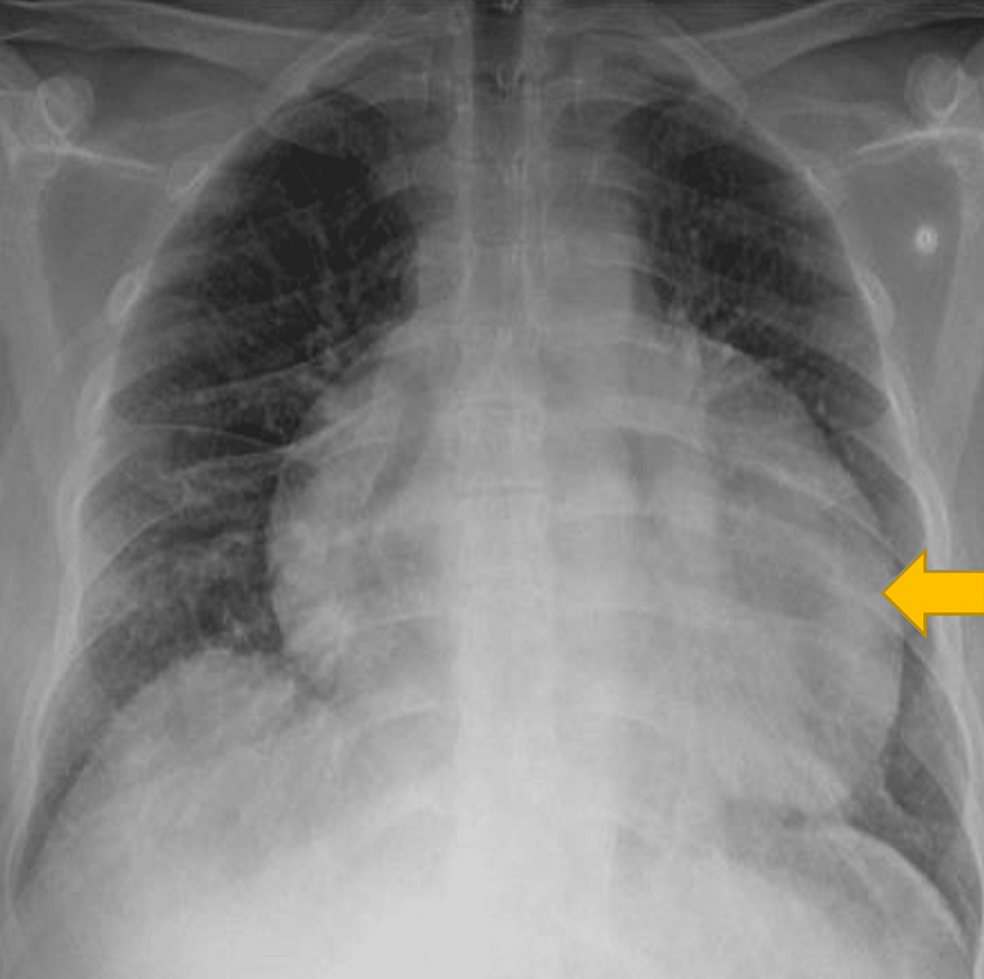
Chest radiography showing a globally increased cardiac shadow in keeping with significant pericardial effusion (yellow arrow).
